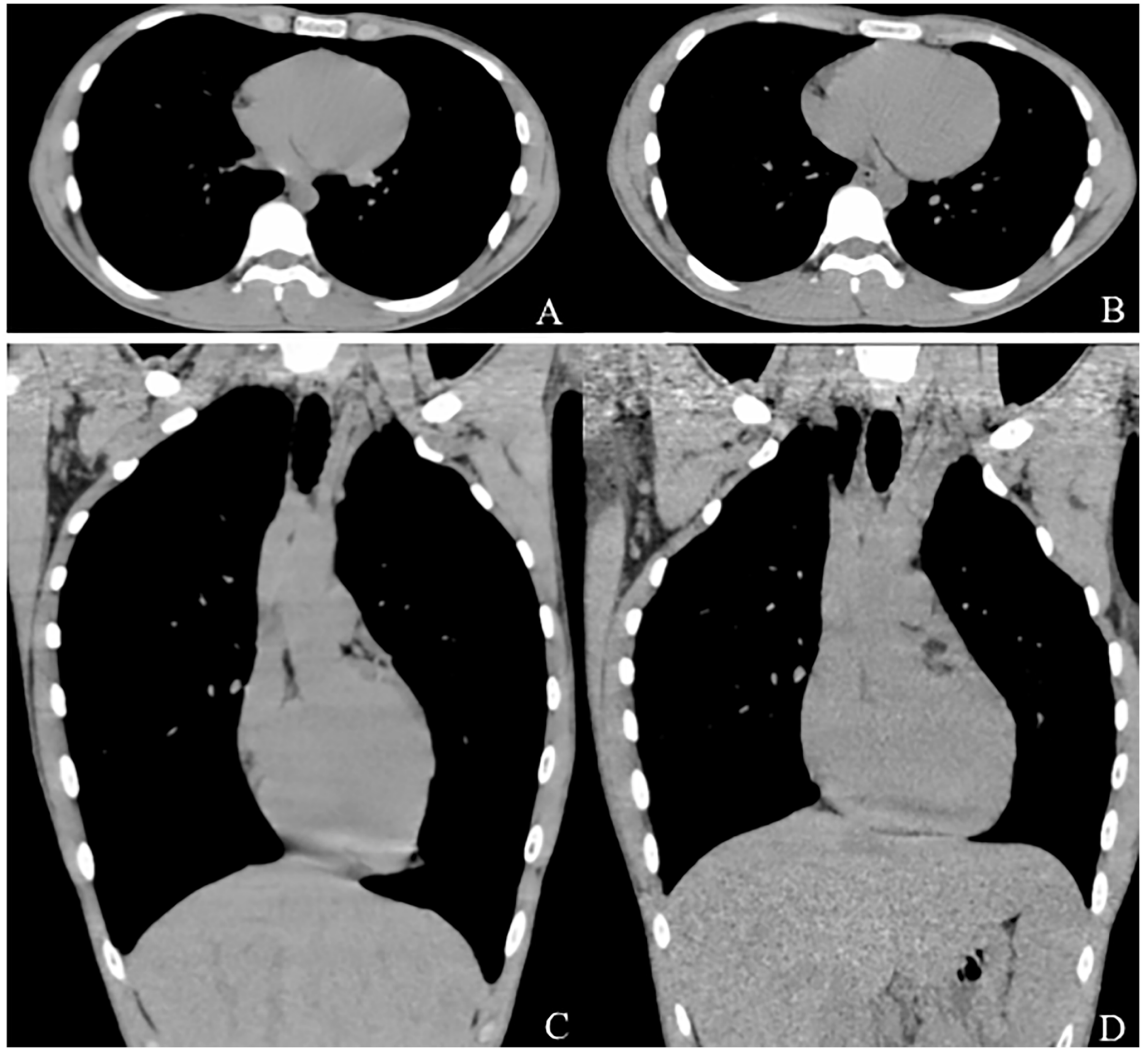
17-year-old male with bronchiectasis. Axial and reconstructed coronal CT images at inspiration (A and C) and at expiration (B and D) are shown. The cardiac cross-sectional area and transverse cardiac diameters are smaller at inspiration (A) than at expiration (B). Based on reconstructed coronal images (C, inspiratory; D, expiratory), the diaphragm is dislocated downward during inspiration and the cardiac long axis leans vertically (C) compared with expiration (D). Note that reconstructed coronal images are shown as a reference and were not used for analysis in the study.
